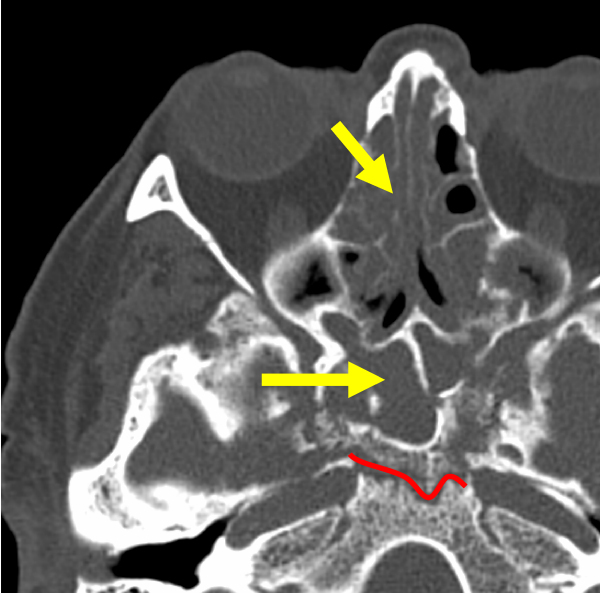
Computed Tomography of the Paranasal Sinuses, Axial Plane, and Bone Window. There is massive content in the ethmoid and sphenoid sinuses (arrows) with a line of sequestration in sphenoid bone (red line).
